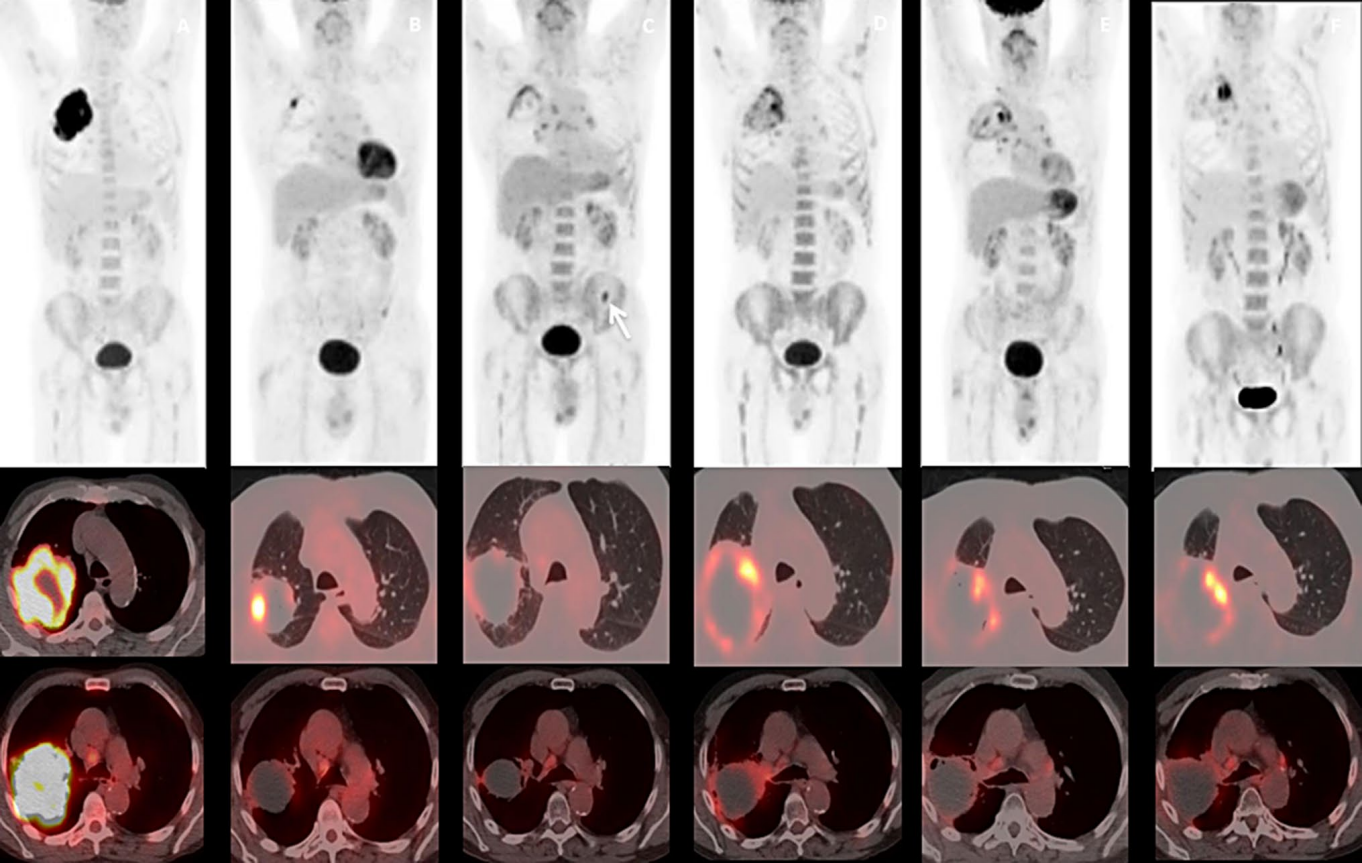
73 year- old male patient diagnosed with NSCLC (wild type EGFR and ALK adenocarcinoma). T3N2 clinical stage. a Basal ^18^F-FDG PET/CT shows hypermetabolic right lung mass with ipsilateral mediastinal involvement. b PET/CT after chemotherapy (Ch) shows partial response. Patient underwent radiotherapy (RT) and second line of Ch. C. PET/CT 3 months after RT shows metabolism in lung mass, probably related with persistence of disease although concomitant inflammation could not be discarded. Hypermetabolic uptake in multiple mediastinal and hilar lymph nodes, probably secondary to inflammation. FDG uptake in left hemipelvis in correspondence with descendent colon (arrow). Colonoscopy demonstrated an adenoma with moderate dysplasia. d Follow-up PET/CT demonstrated progression. Immunotherapy was initiated. e, f PET/CTs during immunotherapy treatment show disease stability
